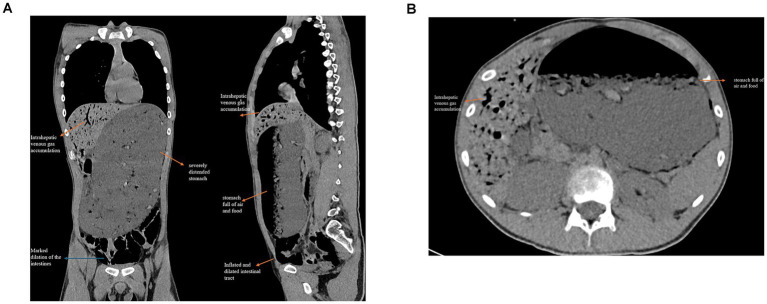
(A,B) The CT image shows a significant dilation of the stomach, with gas accumulation in the esophagus, intestines, and portal vein.
